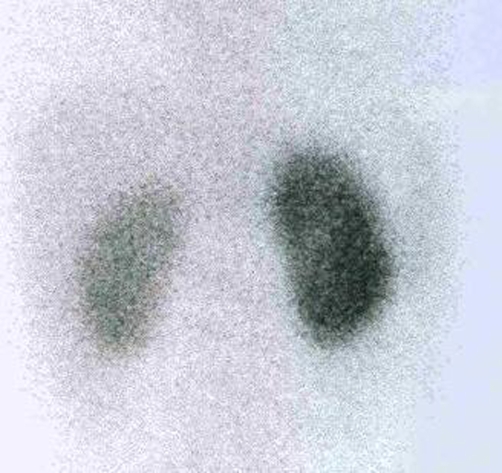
Follow-up ^99m^TC-DMSA renal scintigraphy after enalapril was discontinued. The posterior image shows considerable improvement in the cortical uptake of both kidneys. Right kidney shows normal cortical function, but the left kidney is smaller than the contralateral kidney and its cortical function is less than optimal.
